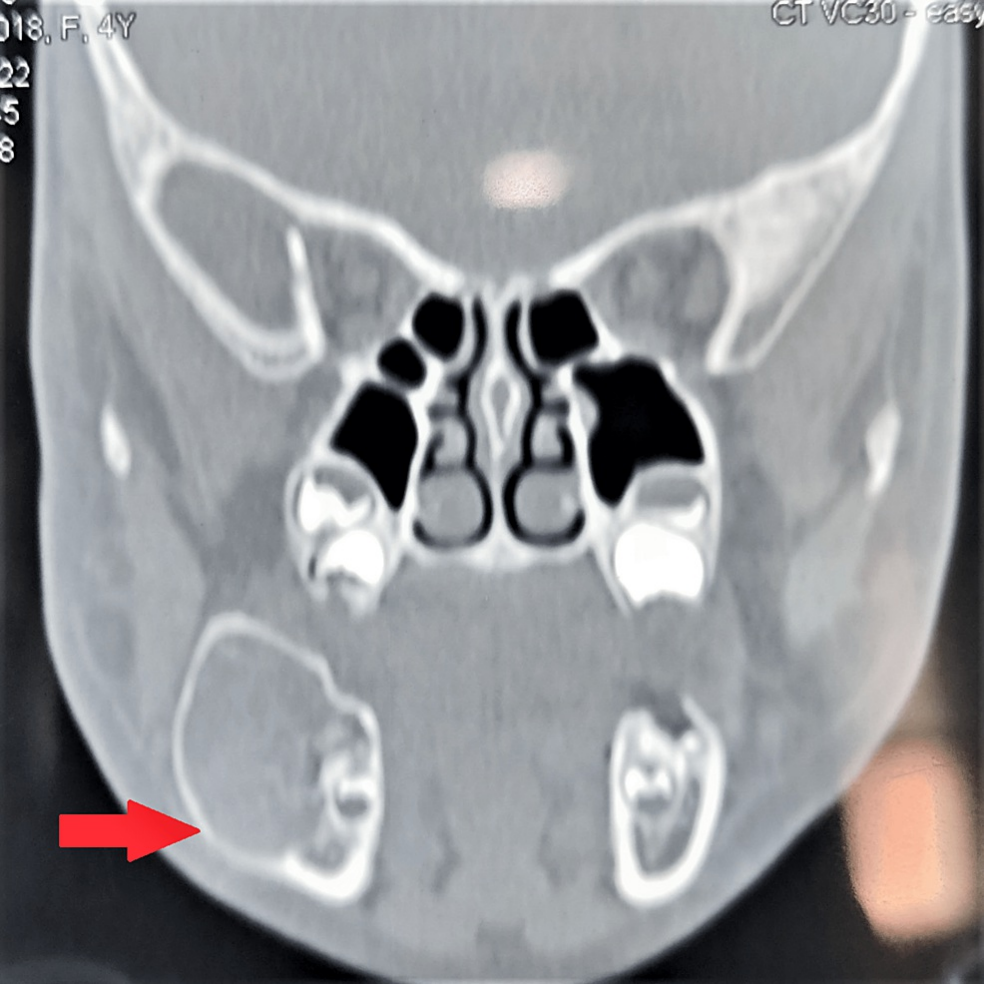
CT face plain coronal section On CT Face plain coronal section bone window, a well-defined expansile lytic lesion was noted arising from the angle of the mandible on the right side extending superiorly till the upper border of the mandible, inferior border of mandible inferiorly. Significant cortical thinning was noted at the perilesional area. No evidence of an obvious cortical breach was noted.
